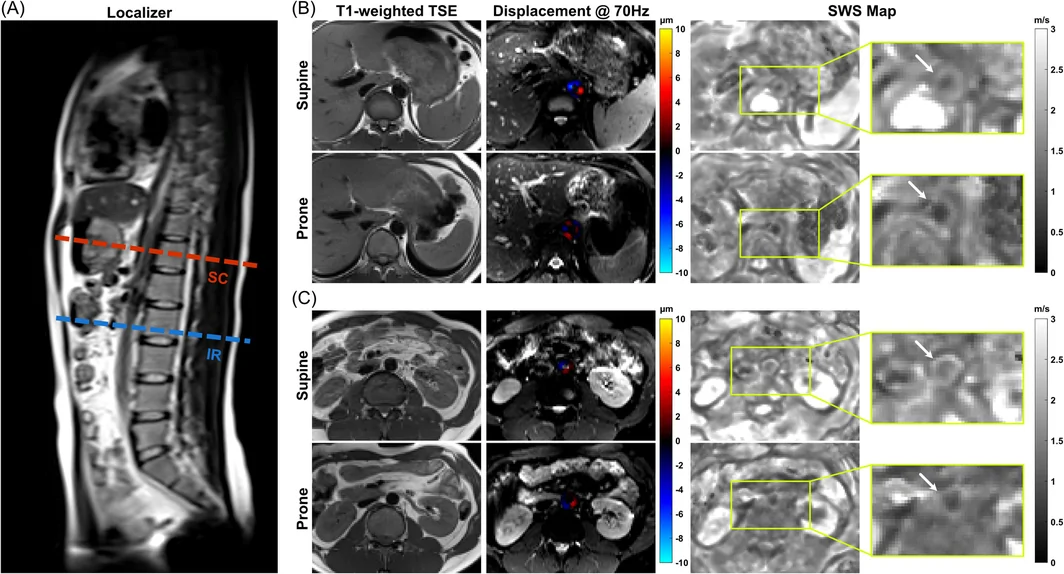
Representative MRI anatomical images as well as MRE magnitude, displacement field, and shear wave speed (SWS) maps of the abdominal aorta are shown in supraceliac (SC) and infrarenal (IR) segments. (A) Sagittal T1‐weighted localizer showing the placement of the two aortic segments (dashed lines): SC (red) above the celiac trunk and IR (blue) below the renal arteries. T1‐weighted turbo spin‐echo (TSE) image, MRE displacement along z‐axis (slice‐select encoding direction) at 70 Hz overlaid on the magnitude images, and MRE SWS map shown in SC (B) and IR (C) segments for supine and prone examination positions.
